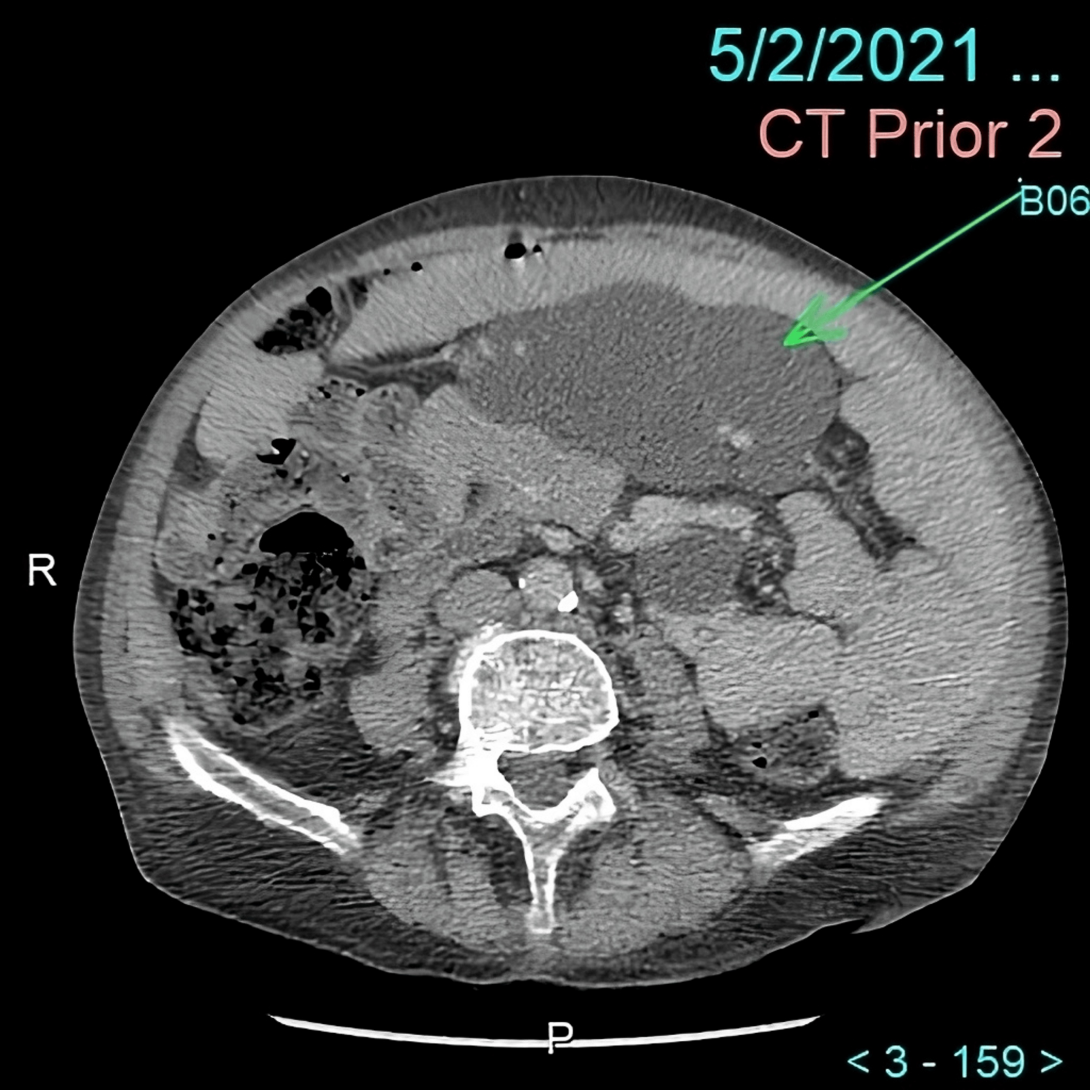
CT abdomen and pelvis acquired after administration of 96 cc IV contrast in May 2021 shows a mesenteric lobulated low attenuating space occupying lesion measuring approximately 12 cm x 6.6 cm with a mean CT number of 11 units (green arrow). There is now bowel obstruction
